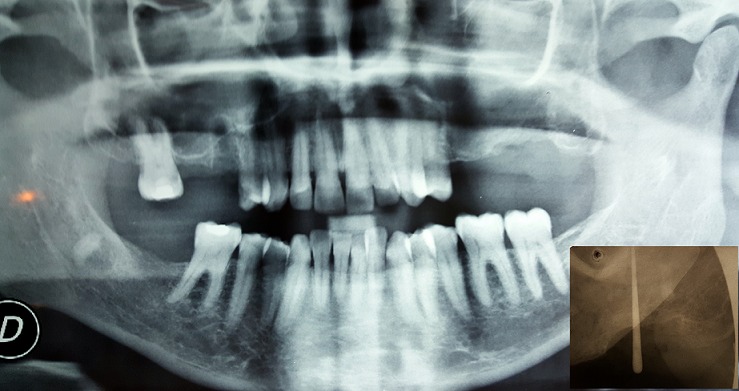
Panoramic x-ray showing an osseous defect in the left upper molar region. The Fistulography bellow confirmed the oroantral communication
